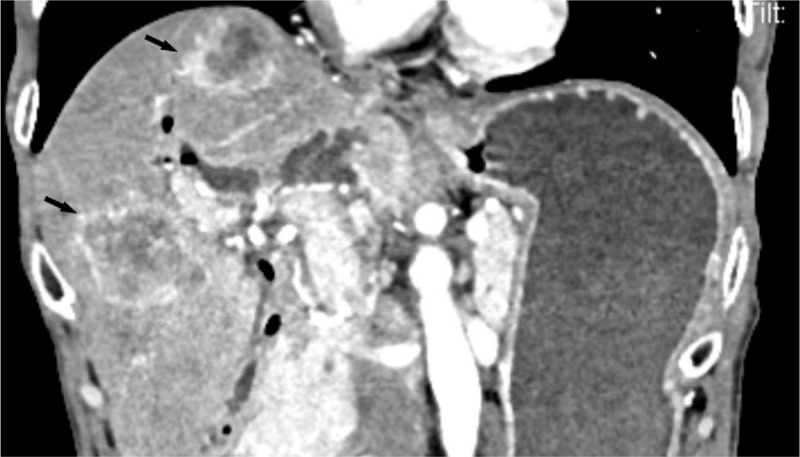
Contrast enhanced computed tomography (CT) scan showed hepatic metastasis (arrow).
